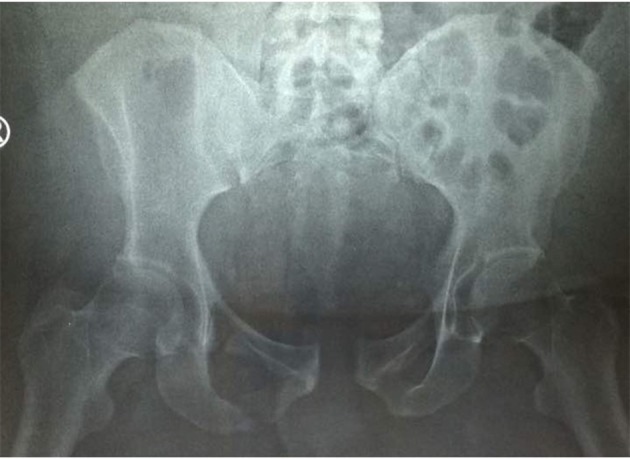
Pelvis AP radiograph of the patient with a BMI of 50 showing unclear findings of sacral bone pathology.
